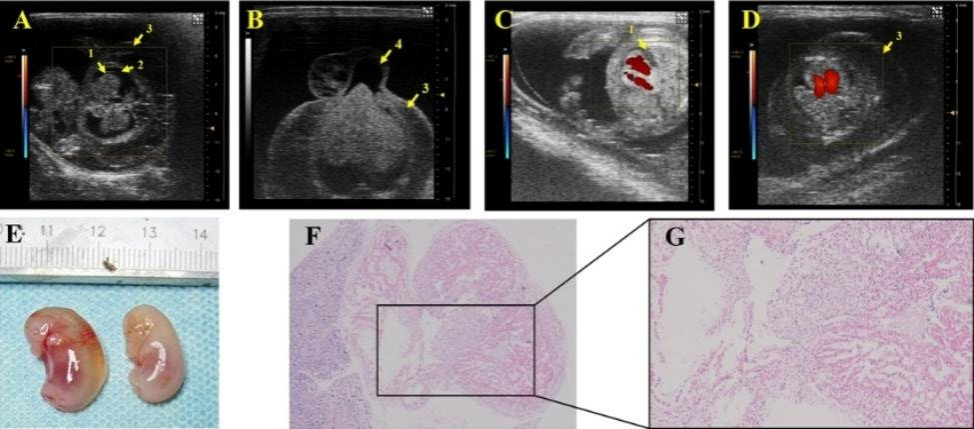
Ultrasonic, anatomical and pathological manifestations of extracardiac lesions. A is a color Doppler image of an aborted fetus with pericardial effusion, pleural effusion and subcutaneous edema. B is a B-mode image of a fetus with umbilical hernia and subcutaneous edema. C is a color Doppler image of a viable fetus with pleural effusion. D is a color Doppler image of a viable fetus with subcutaneous edema. E is the comparison of a fetus with subcutaneous edema (left) to a fetus with a normal appearance from the same litter (right). F and G are pathology images of the fetus with edema (magnifications are 40× and 100×, respectively), indicating VSD, loose heart tissue, and an incompletely formed heart chamber. (Arrow 1 indicates pericardial effusion, arrow 2 indicates pleural effusion, arrow 3 indicates subcutaneous edema, and arrow 4 indicates umbilical hernia.)
